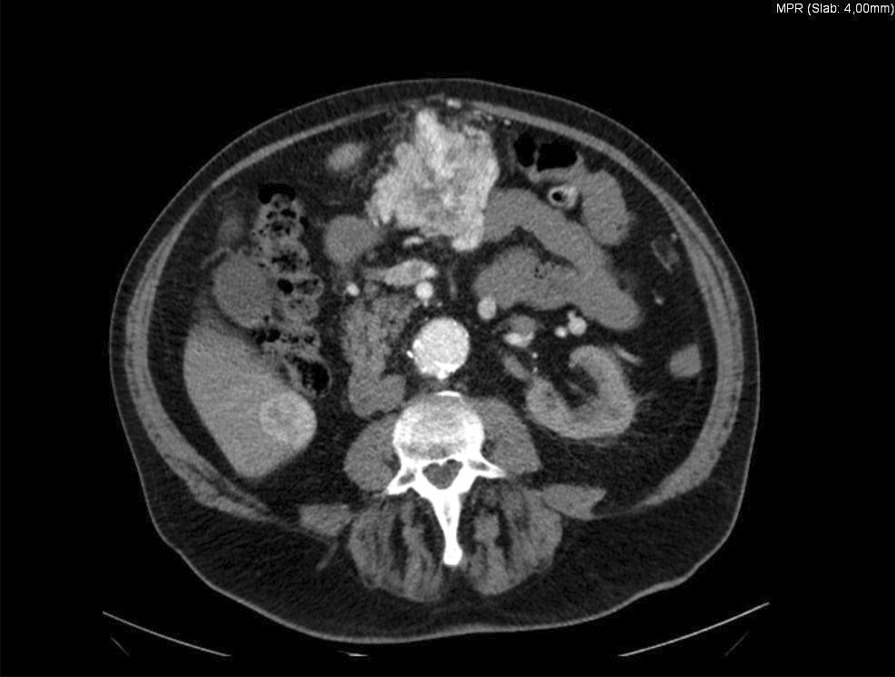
Arterial phase of CT scan showing hypervascular tumor in a right caudal liver segment as well as intraperitoneal in the upper abdomen
